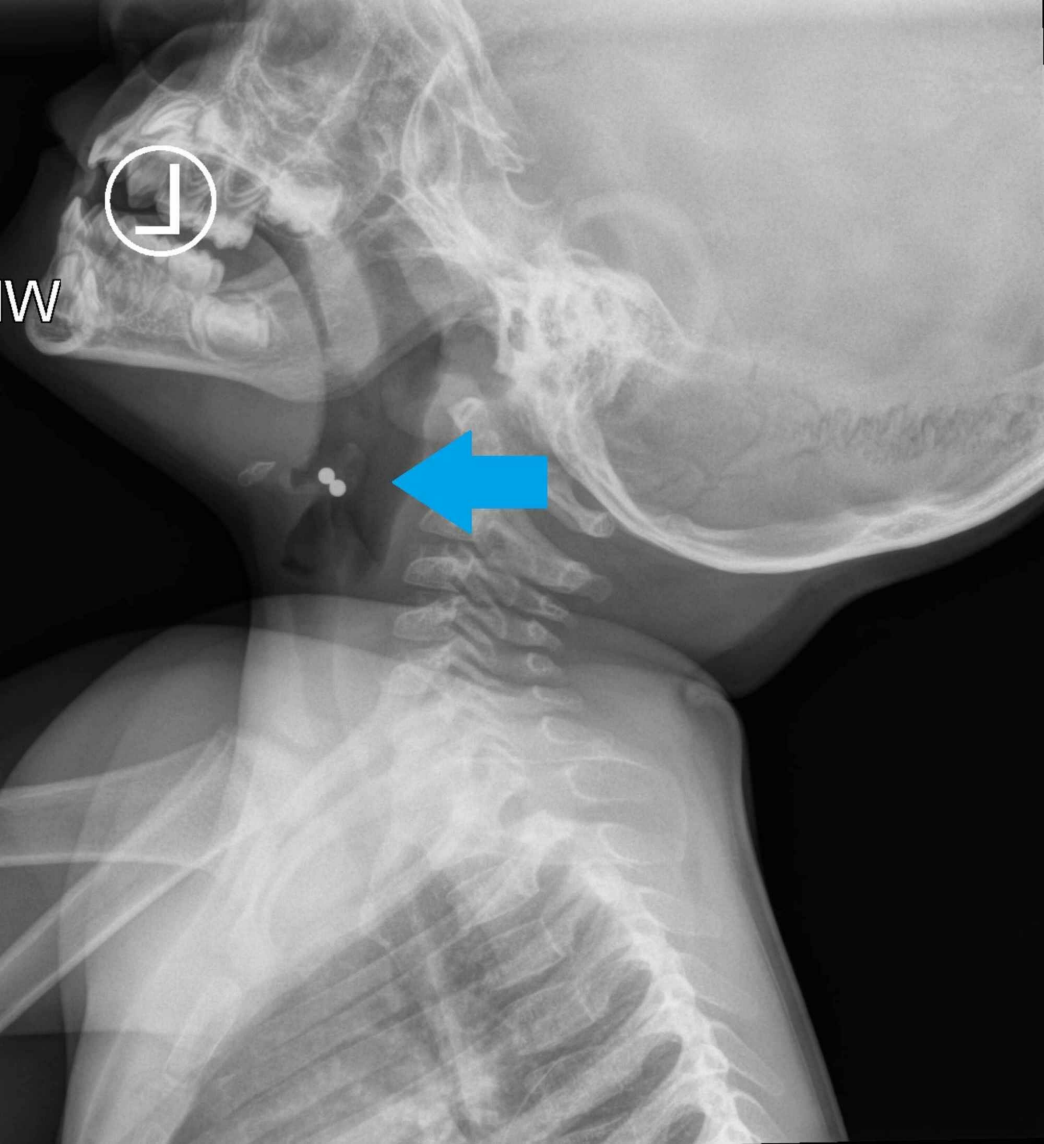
Plain film of the neck in a two-year-old that ingested neodymium ball magnets that are located at the level of the epiglottis
